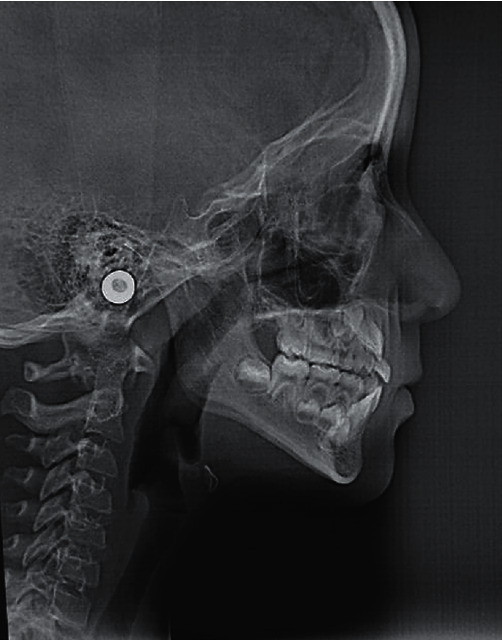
The lateral cephalic radiography shows skeletal Class III due to mild maxillary macrognathism.
